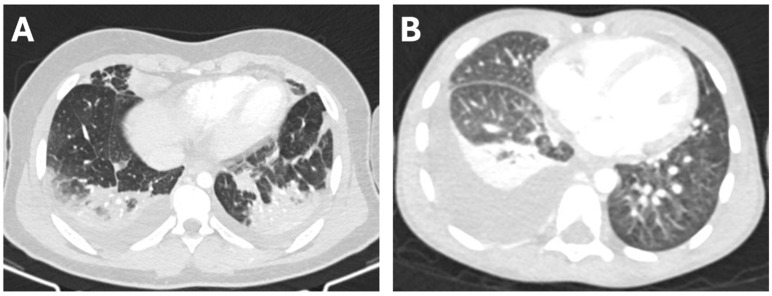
Transverse chest CT scans from a 13-year-old boy with PEf associated with parainfluenza virus pneumonia (A), and from a 5-year-old boy with PEm associated with pneumococcal pneumonia (B).
